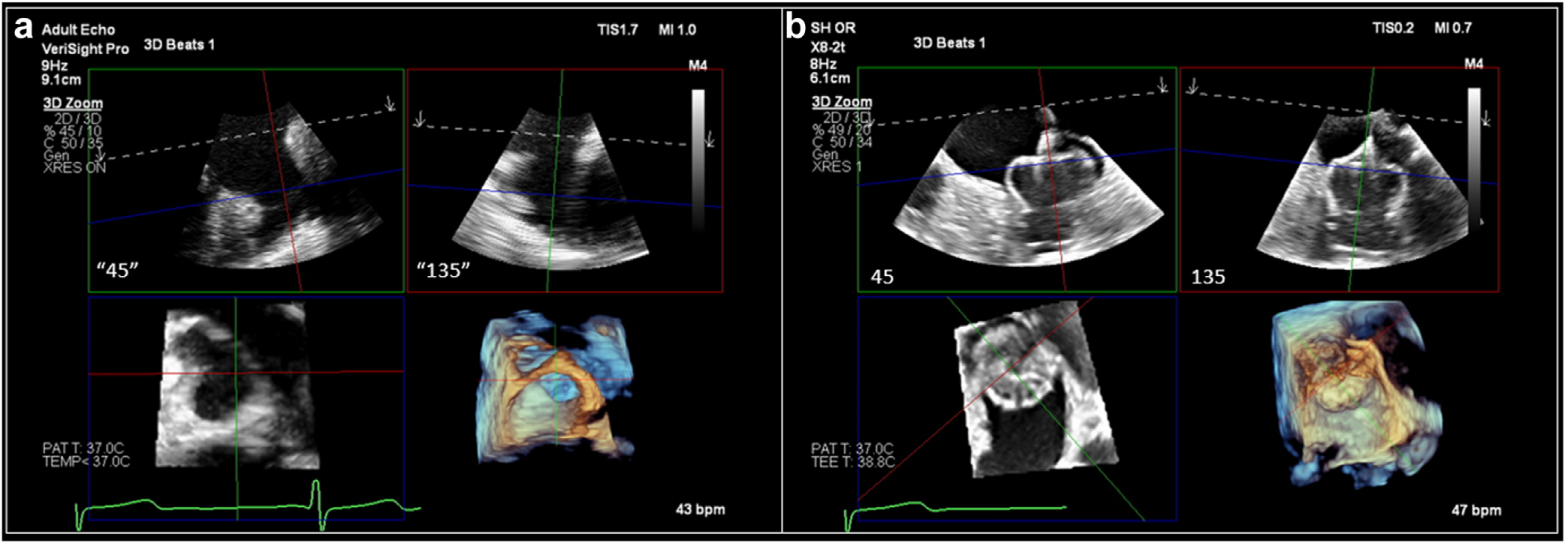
ICE image analysis using 3D MPR to mimic traditional TEE views. Panel A: baseline LAA images from the mid-LA vantage point manipulated to mirror traditional TEE views. Panel B: postimplant TEE images demonstrating identical anatomy to baseline ICE images with a well-seated Watchman device in place Abbreviations: 3D, three-dimensional; ICE, intracardiac echocardiography; LA, left atrial; LAA, left atrial appendage; MPR, multiplanar reconstruction; TEE, transesophageal echocardiography.
